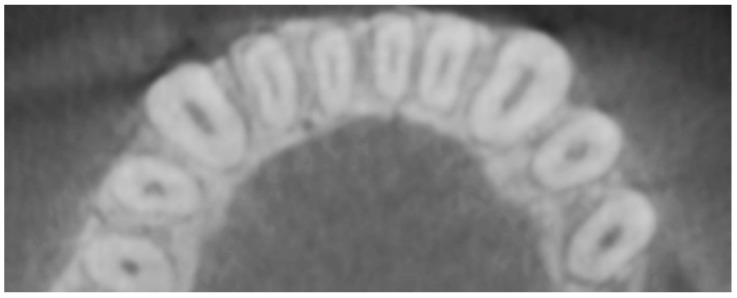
Cone Beam Computed Tomography (CBCT) image of modified piezocision corticotomy between 4-2 and 4-3.
